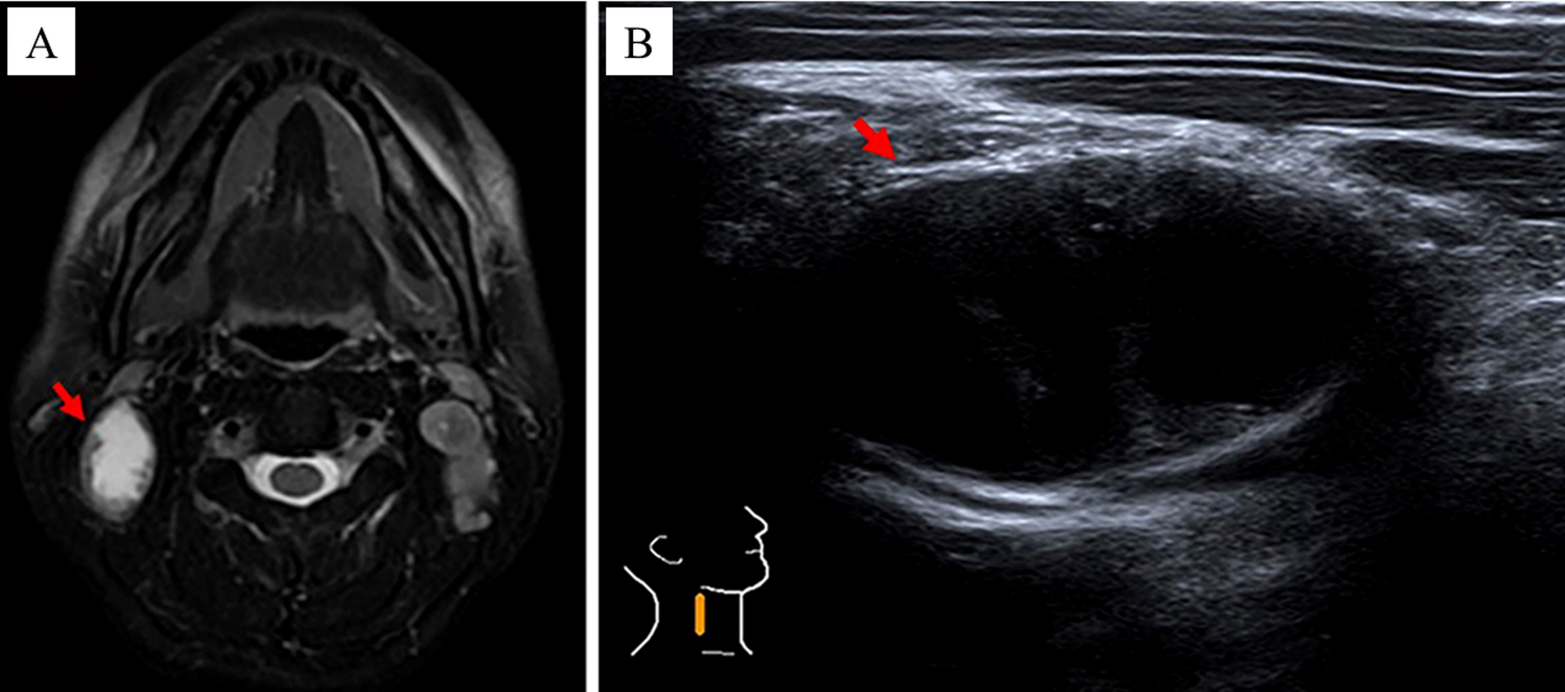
The method of locating the tCNN. In a 37-year-old male NPC patient, by referring to (A) MRI images, the (B) two-dimensional ultrasound was performed to locate the tCNN, as indicated by the red arrow.
